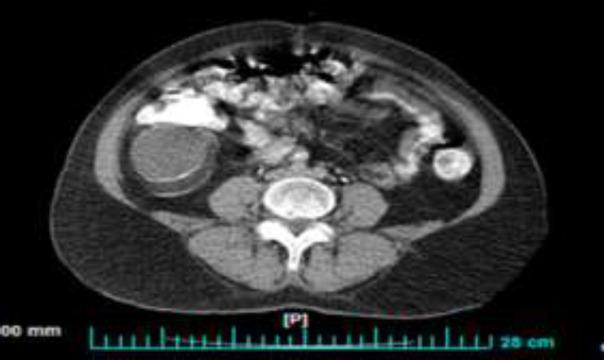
Abdomino-pelvic CT scan demonstrating a lobulated tubular calcified mass-like lesion with peripheral calcification in right lower quadrant
